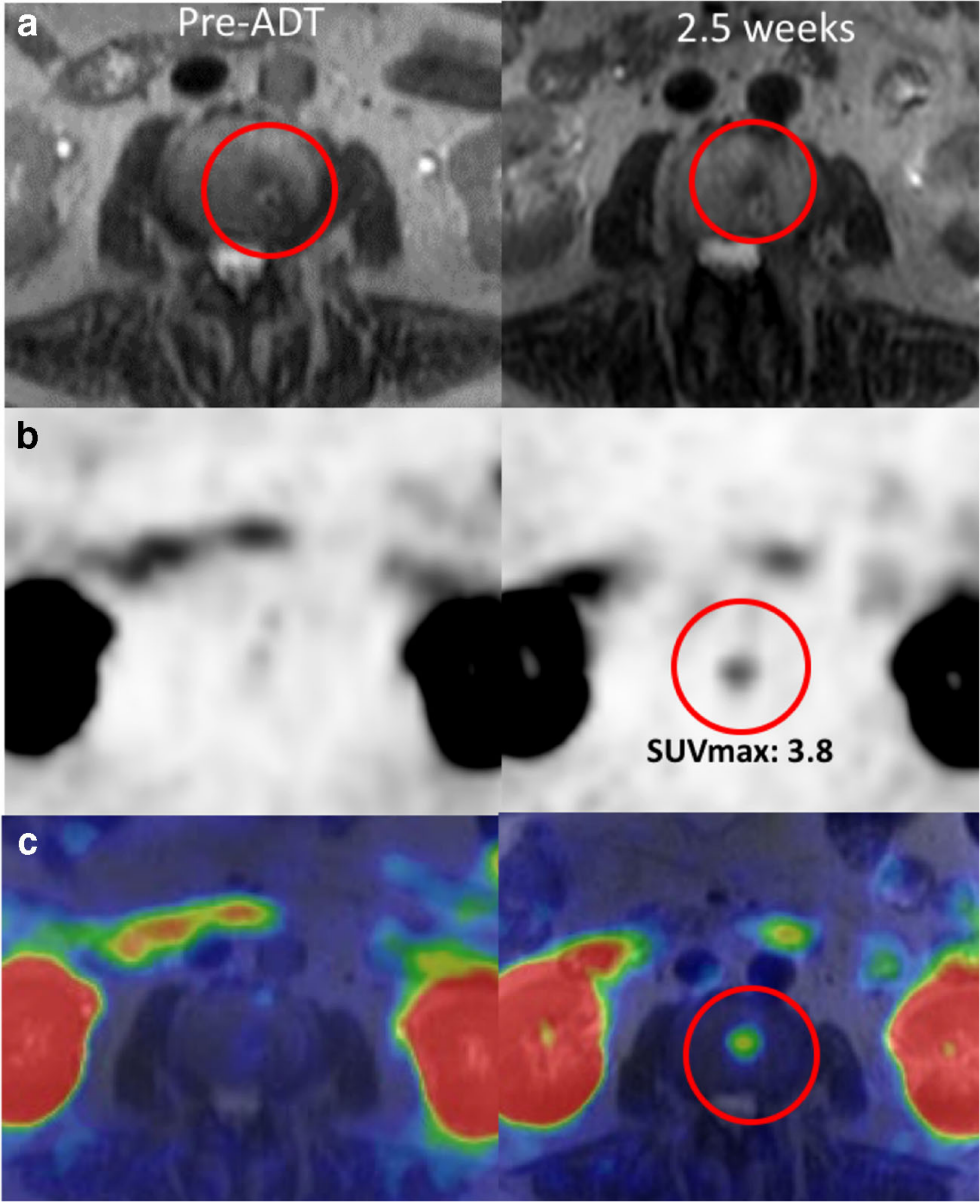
T2W-MRI (a), PET (b), and fused PET/MRI (c) images of patient no. 8 at baseline and 2.5 weeks after ADT (degarelix). A new bone metastasis in L2 vertebra was detected. The lesion was already visible at baseline T2W-MRI images but PSMA uptake occurred 2.5 weeks after the initiation of ADT
